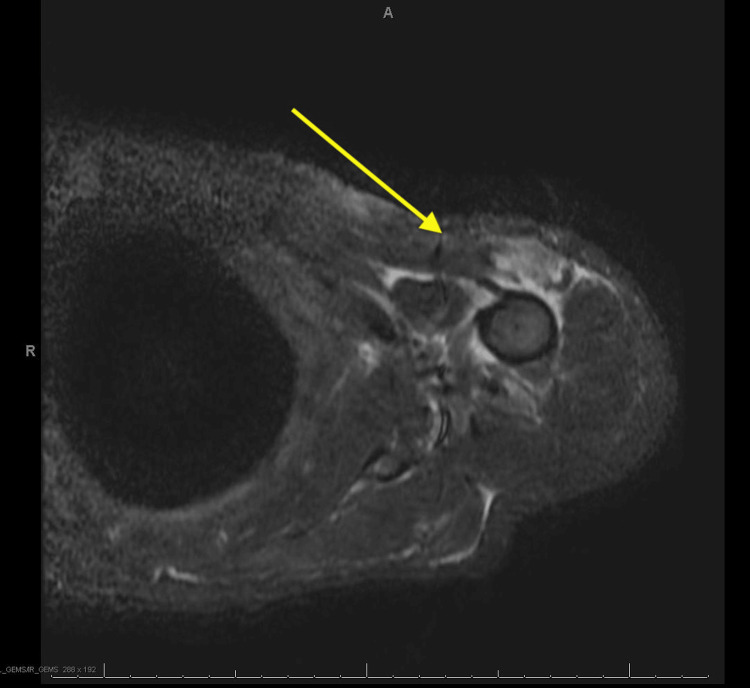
The MRI of the left shoulder showing a significant sinus tract (arrow) from the shoulder wound to the left subdeltoid bursa.
